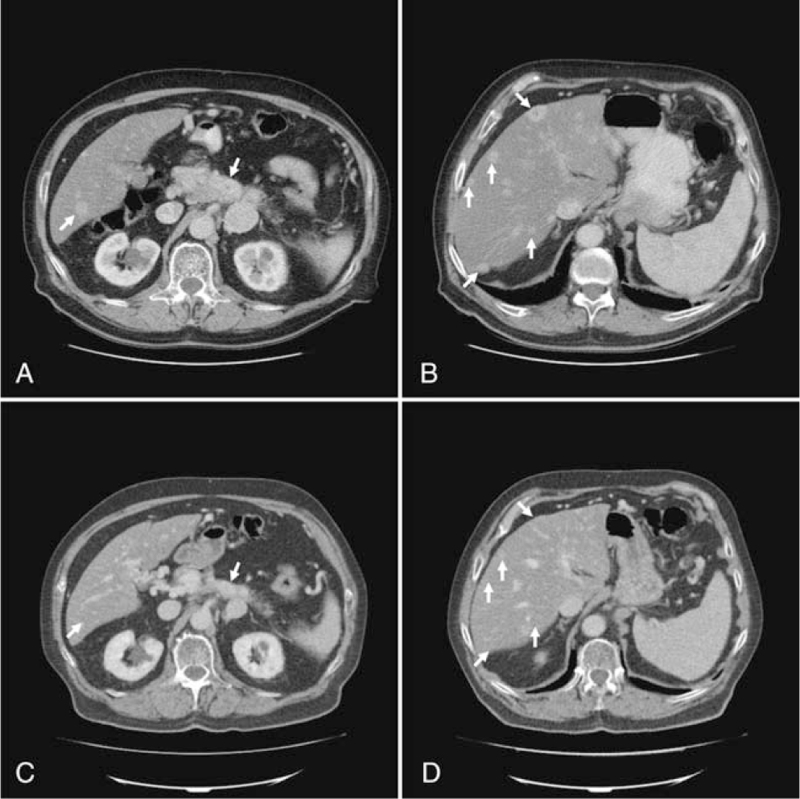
Initial and follow-up imaging of Case 1. (A) The initial contrast-enhanced CT of the abdomen detected a hyperdense lesion within the liver and the pancreatic corpus (white arrow). (B) The initial contrast-enhanced CT imaging demonstrated multiple hyperdense lesions in both liver lobes (white arrows). (C) The follow-up CT showed partial response of the initial hyperdense lesion within the pancreatic corpus after induction of systemic chemotherapy according STZ/5-FU (white arrow). (D) The follow-up CT scan confirmed a partial remission of the known hyperdense hepatic lesions after induction of systemic chemotherapy according STZ/5-FU (white arrows). 5-FU = 5-fluorouracil, CT = computed tomography, STZ = streptozocin.
